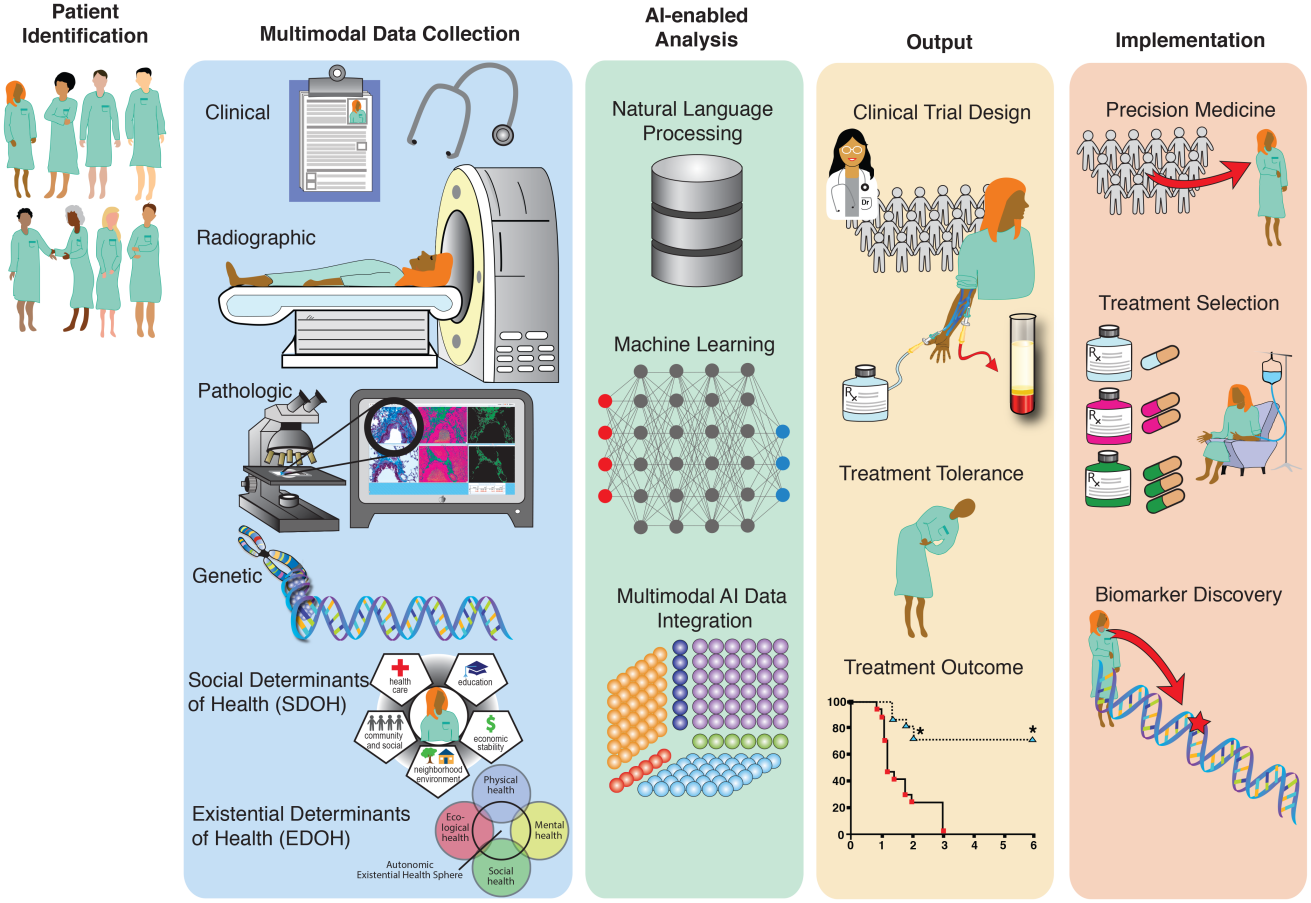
Ongoing and Potential Integrations of AI in Oncology. There is increasing efforts to combine clinicopathologic factors, social and existential determinants of health, and radiographic features using AI approaches to evaluate cancer therapy treatment outcomes and improve cancer care.
